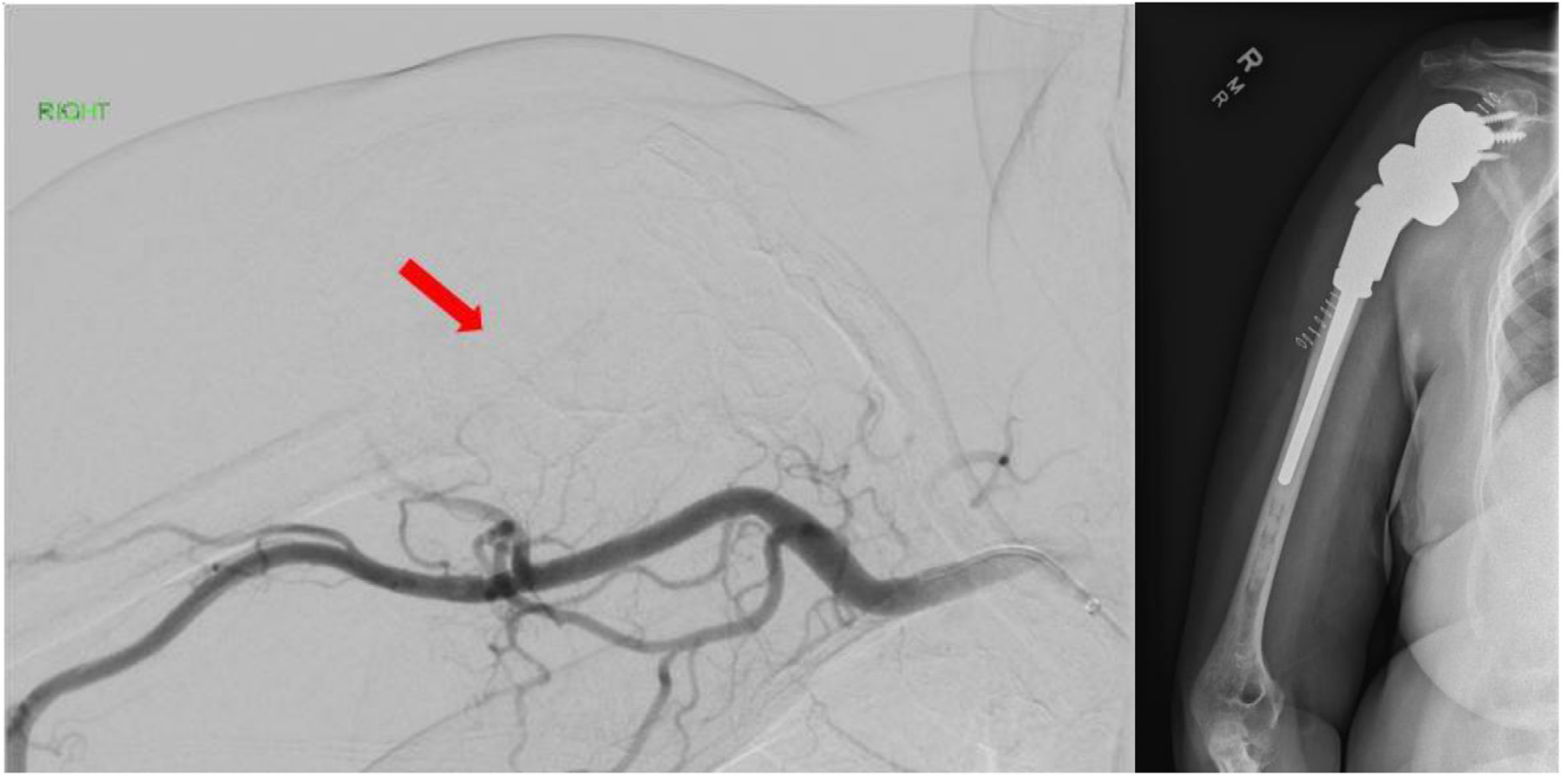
(A) Postembolization right subclavian artery DSA demonstrates cessation of flow to the tumor. (B) X-ray image of right total shoulder arthroplasty using a long intramedullary rod component.
